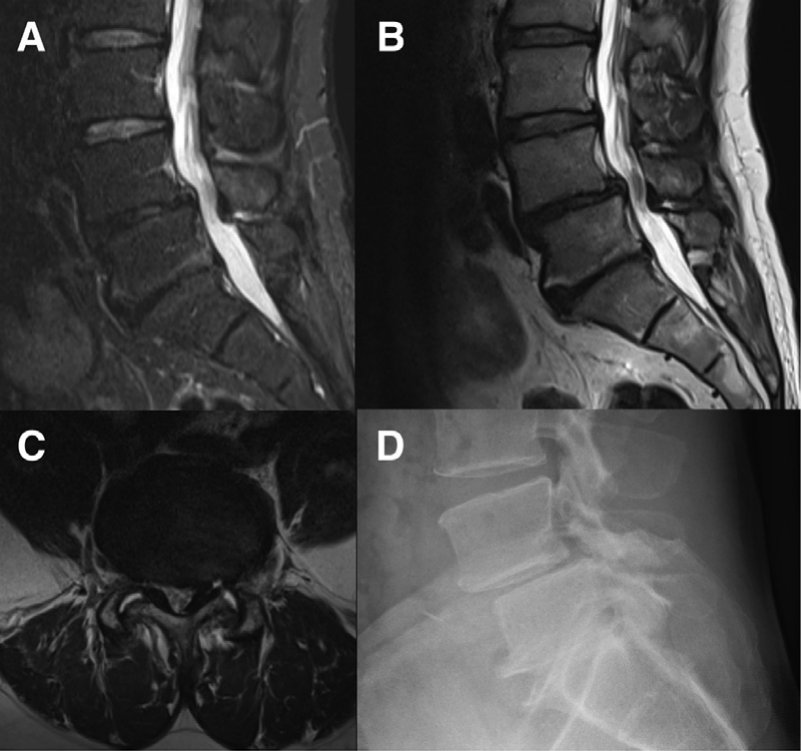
Preoperative images: (A) sagittal fat suppressed MRI (turbo inversion recovery magnitude (TIRM)) and (B) sagittal MRI (T2 sequence) demonstrating segment degeneration at L4/5 and L5/S1, (C) axial MRI (T2 sequence) at level L4/5 showing spinal stenosis, (D) lateral radiograph showing accentuated spondylolisthesis at L4/5 in standing position.
